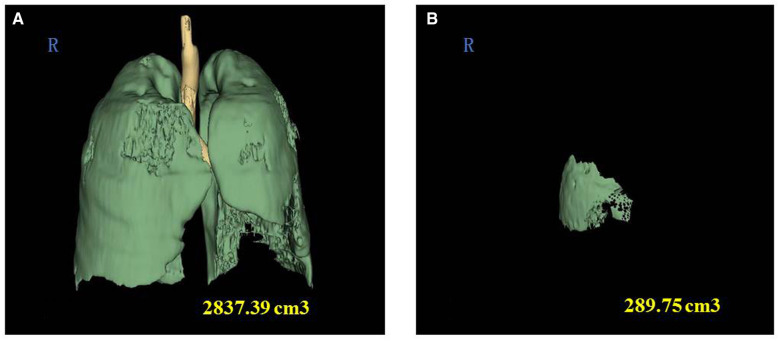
Three-dimensional reconstruction of chest CT images to calculate the volume fraction of pulmonary contusion. (A) Three-dimensional reconstruction of chest CT images showing the total volume of pulmonary contusion. (B) Three-dimensional reconstruction of chest CT images of a 61-year-old female patient showing the volume of pulmonary contusion. The volume fraction of pulmonary contusion = 289.75 cm^3^/2,837.39 cm^3^ *100% = 10.2%.
